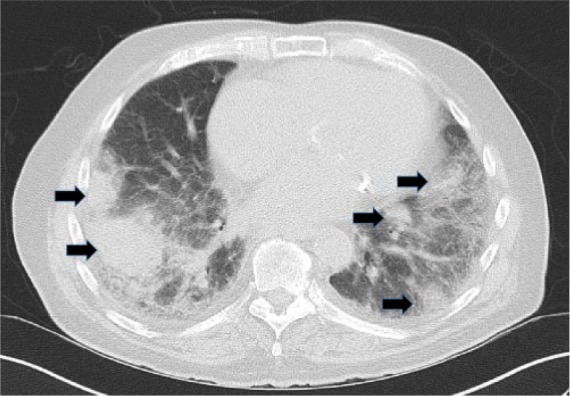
Computed tomogram of the chest, axial cuts with arrows pointing at the multiple nodular densities through the lung fields bilaterally.
